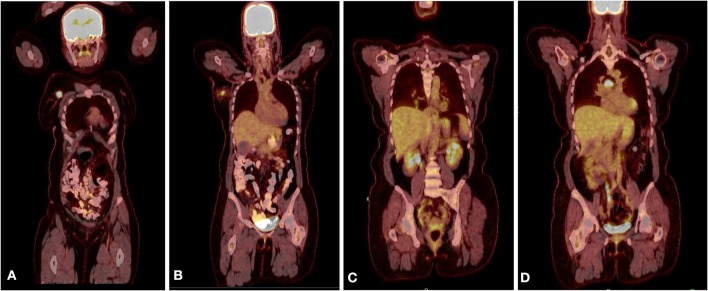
(A) PET CT scan showing the primary lesion in the right upper outer quadrant of the right breast. (B) A hyper metabolic right axillary lymph node, which is compatible with the patient's known right breast primary malignancy and a presumed metastatic right axillary lymph node. (C) PET scan after recurrence showing new lung nodules with FDG uptake. (D) PET scan after recurrence showing sub-carinal lymph node with new intense focus of FDG uptake.
